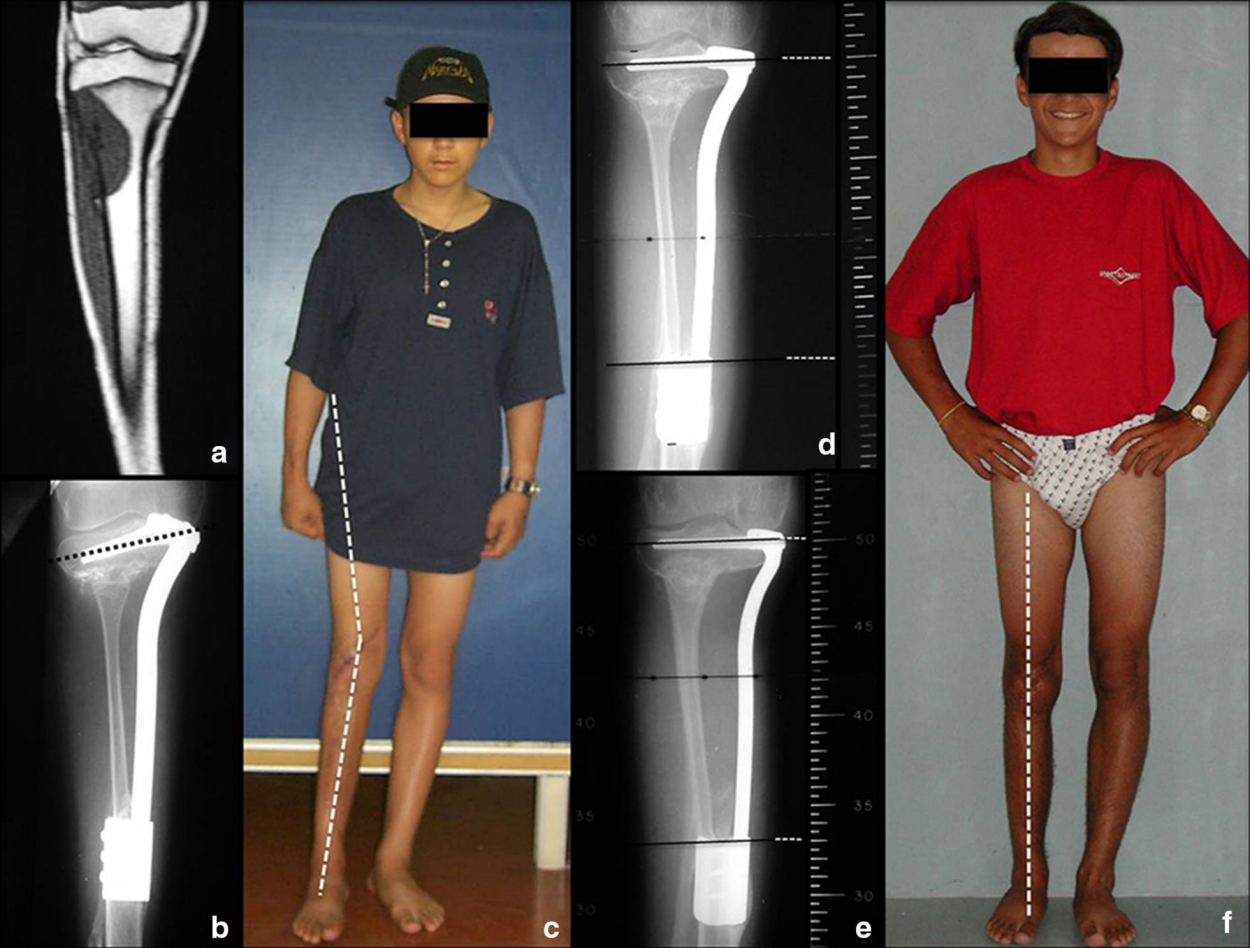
a Preoperative magnetic resonance, b 4 months postoperative radiograph showing the slope of the tibial epiphysis screws, c patient at 4 months after surgery with valgus knee deviation, d patient at 1 year and 2 months after surgery, with fixed valgus deformity and e 1 year and 2 months postoperative radiograph showing fibula hypertrophy, screws tilt correction and growth of 0.75 cm and f radiography postoperative 2 years and 2 months with growth of 1.2 cm
